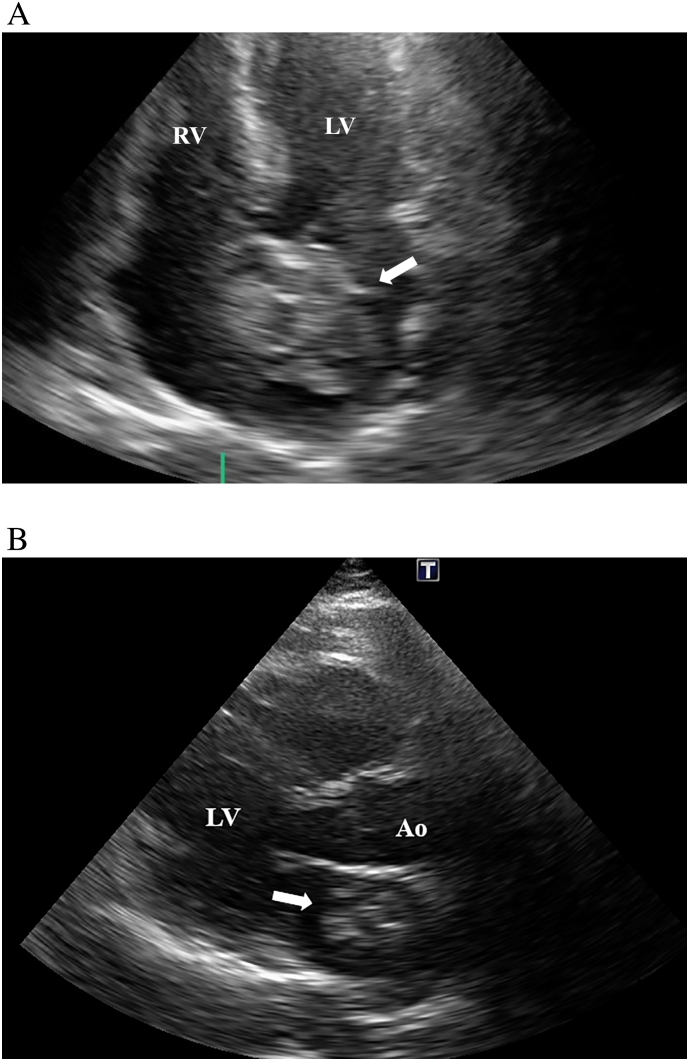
A. Transthoracic echocardiography imaging shows a large left atrial thrombus with calcification attached to the atrial septum (4 chamber view). B. Transthoracic echocardiography imaging shows a large left atrial thrombus with calcification attached to the atrial septum (long axis view).
